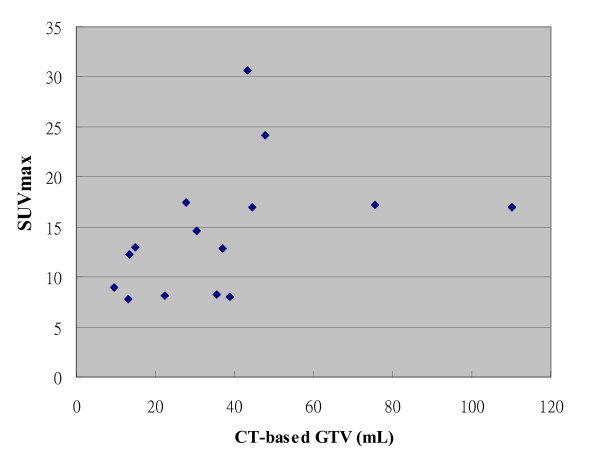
The association between the SUVmax and the CT-based pGTV.
